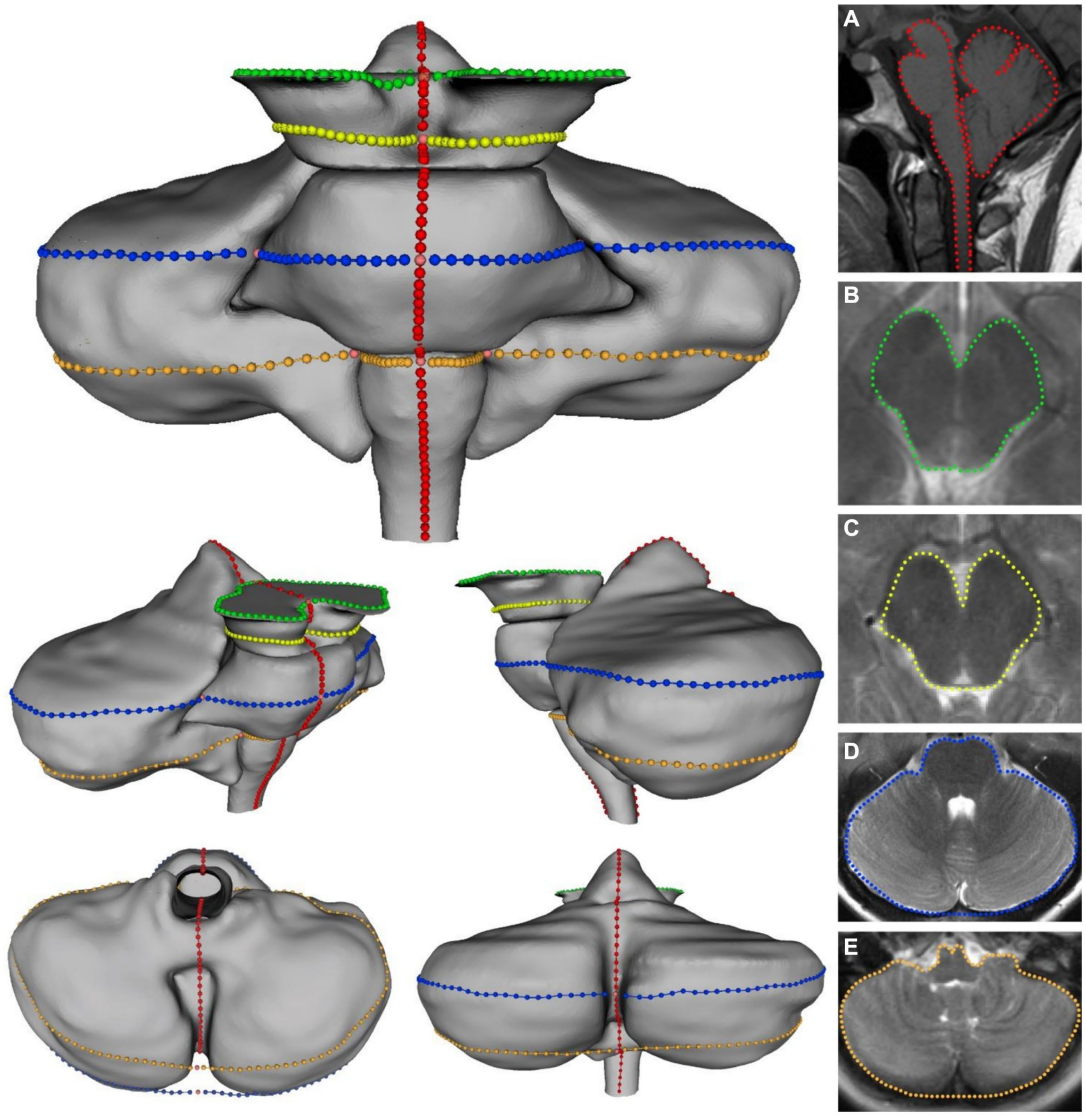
Visualization of the five landmarking protocols in 3D with corresponding 2D image for: (A) Midsagittal brainstem and cerebellum; (B) superior midbrain; (C) inferior midbrain; (D) pons/cerebellum; (E) medulla/cerebellum. Colors on 3D model correspond with colors in MRI images.
